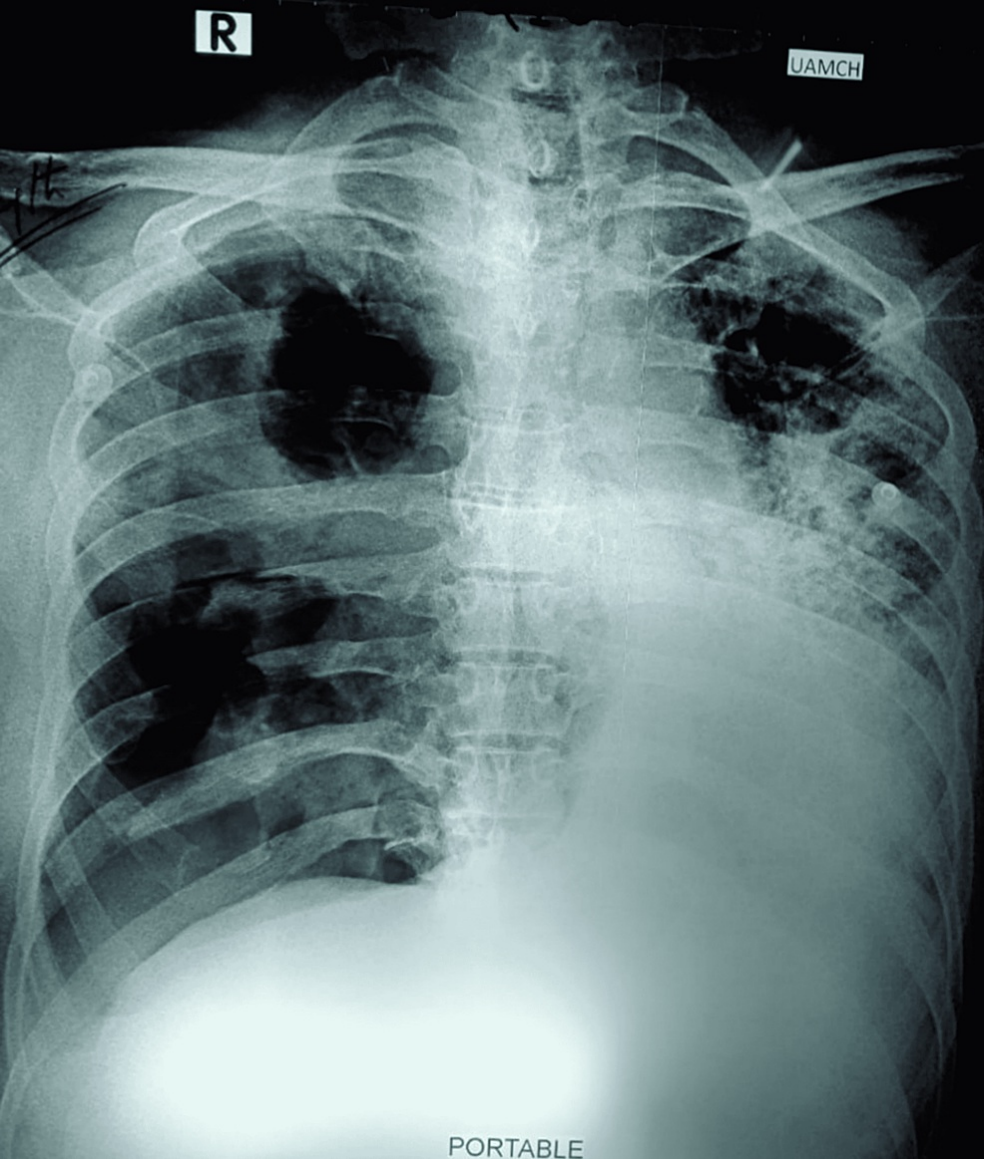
Portable chest X-ray revealing bilateral consolidation with left-sided effusion.
